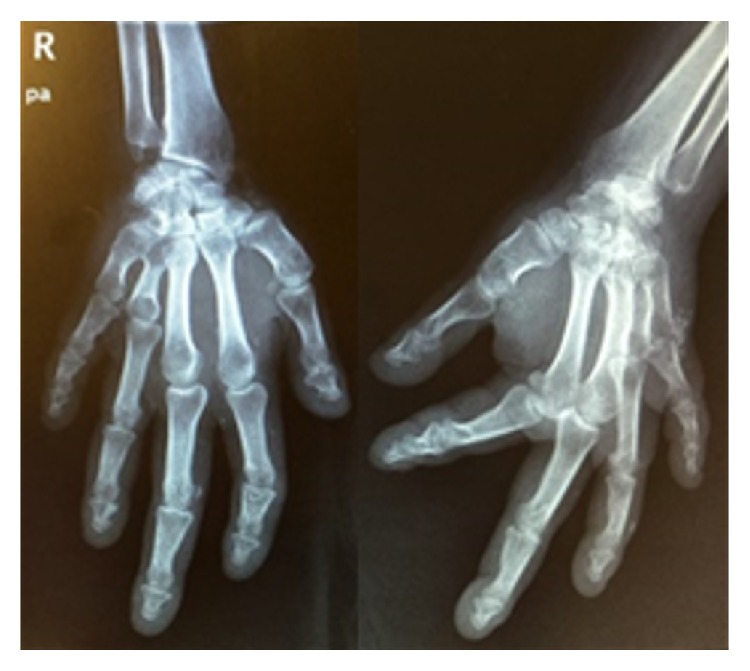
Radiography of both hands, revealing brachydactyly of the fourth and fifth metacarpals.
